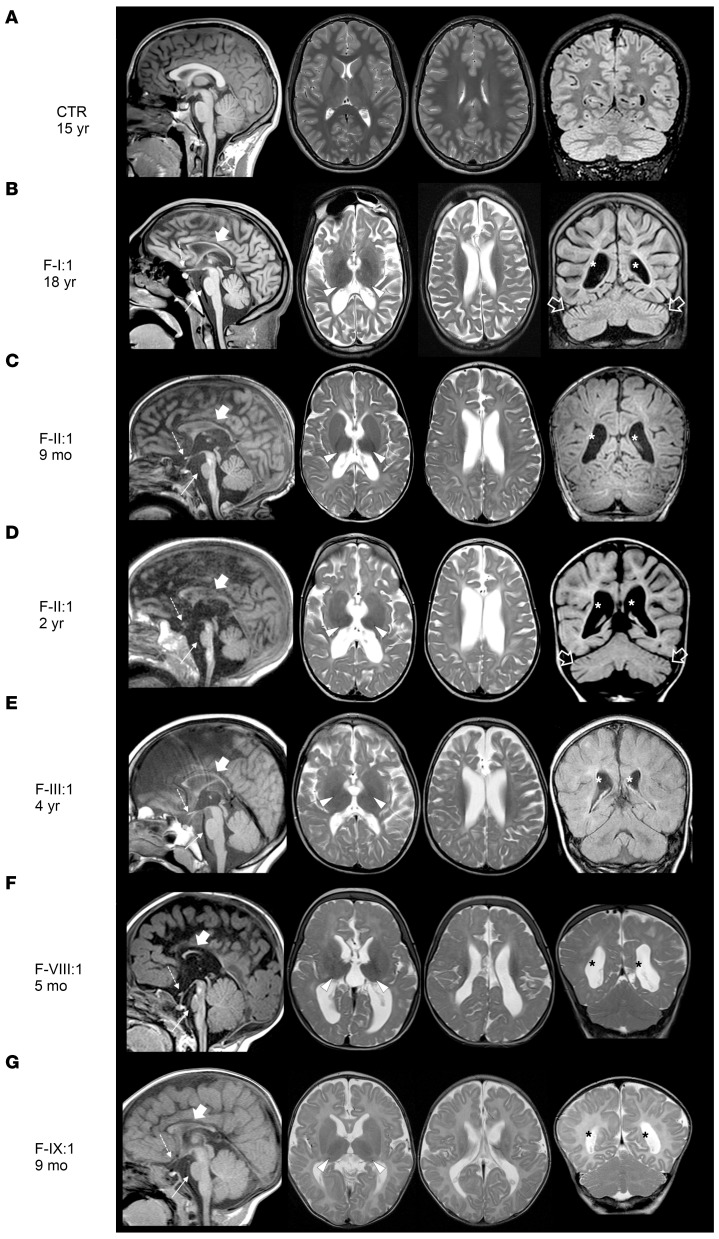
Neuroimaging findings in individuals with *BORCS5* missense and splice-site variants. Brain MRI studies of a control individual performed at 15 years of age for comparison (A) and of individuals F-I:1 (B), F-II:1 (C and D), F-III:1 (E), F-VIII:1 (F), and F-IX:1 (G). Presented are sagittal T1-weighted images (first column), axial T2-weighted images (second and third column), and coronal FLAIR or T1/T2-weighted images (last column). There is moderate to severe cerebral atrophy and loss of white matter volume with consequent ventricular dilatation in all individuals (marked with *). The myelination is markedly reduced or incomplete in all cases. The corpus callosum is very thin in all individuals (thick arrows) with associated hypoplastic anterior commissure. In F-VIII:1 and F-IX:1, the corpus callosum is also short and dysplastic. There is atrophy of the optic nerves (not shown) and chiasm (dashed arrows) in all patients. The thalami are small and hypointense on T2-weighted images (arrowheads). The midbrain and pons are small in all individuals (thin arrows), especially F-II:1 and F-VIII:1. Mild atrophy is noted in F-I:1 and F-II:1 (empty arrows). Note the clear progression of cerebral and cerebellar atrophy with arrested myelination in FII:1 (C and D).
